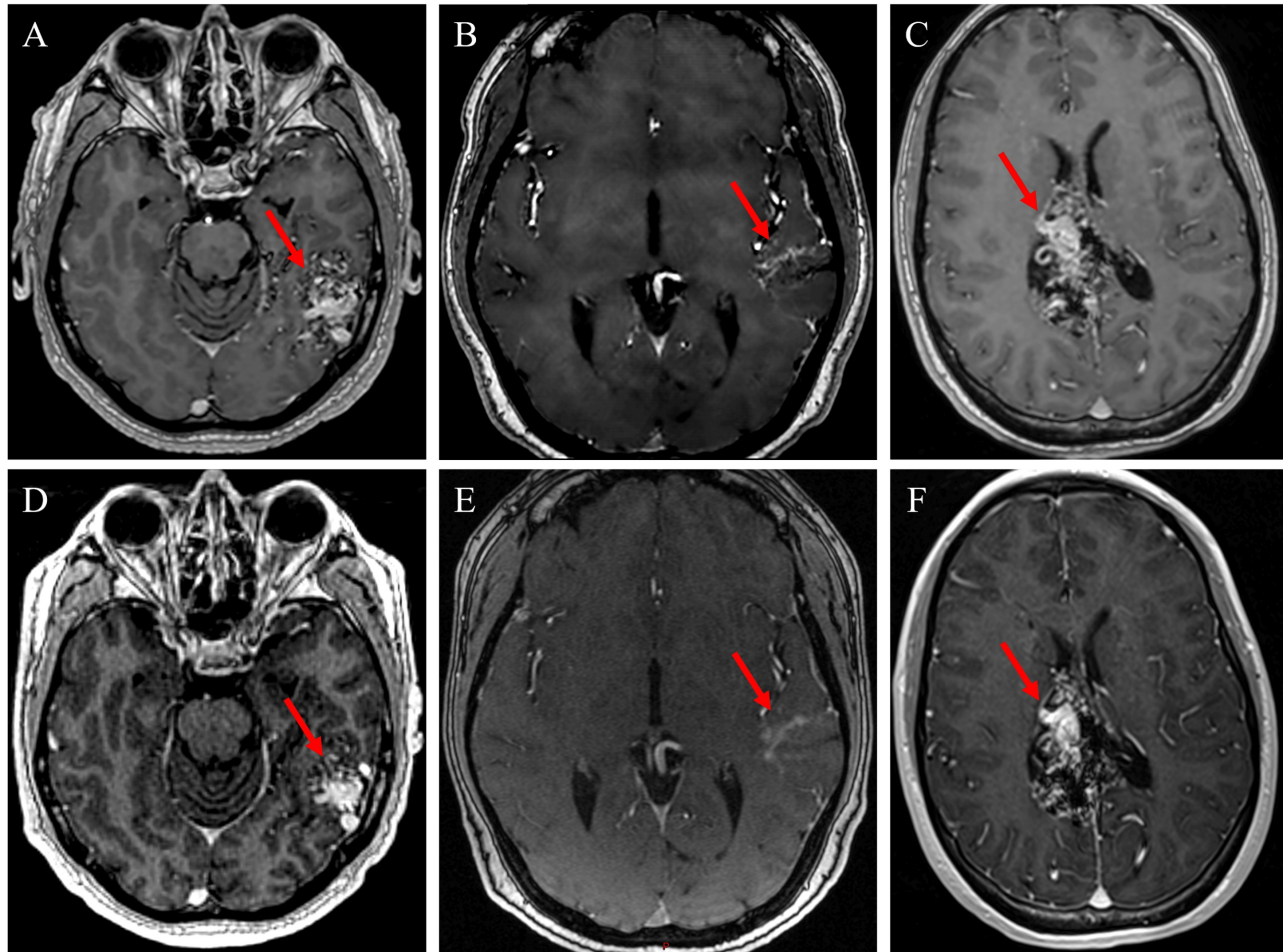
Comparison of pre- and post-treatment MRIs: A) Patient 1: pre-treatment T1 MRI, B) patient 2: pre-treatment MRA, C) patient 3: pre-treatment T1 MRI, D) patient 1: one-year post-treatment T1 MRI, E) patient 2: four-month post-treatment MRA, F) patient 3: six-month post-treatment T1 MRI. Image Credit: Michael Chaga MRA, magnetic resonance angiography
